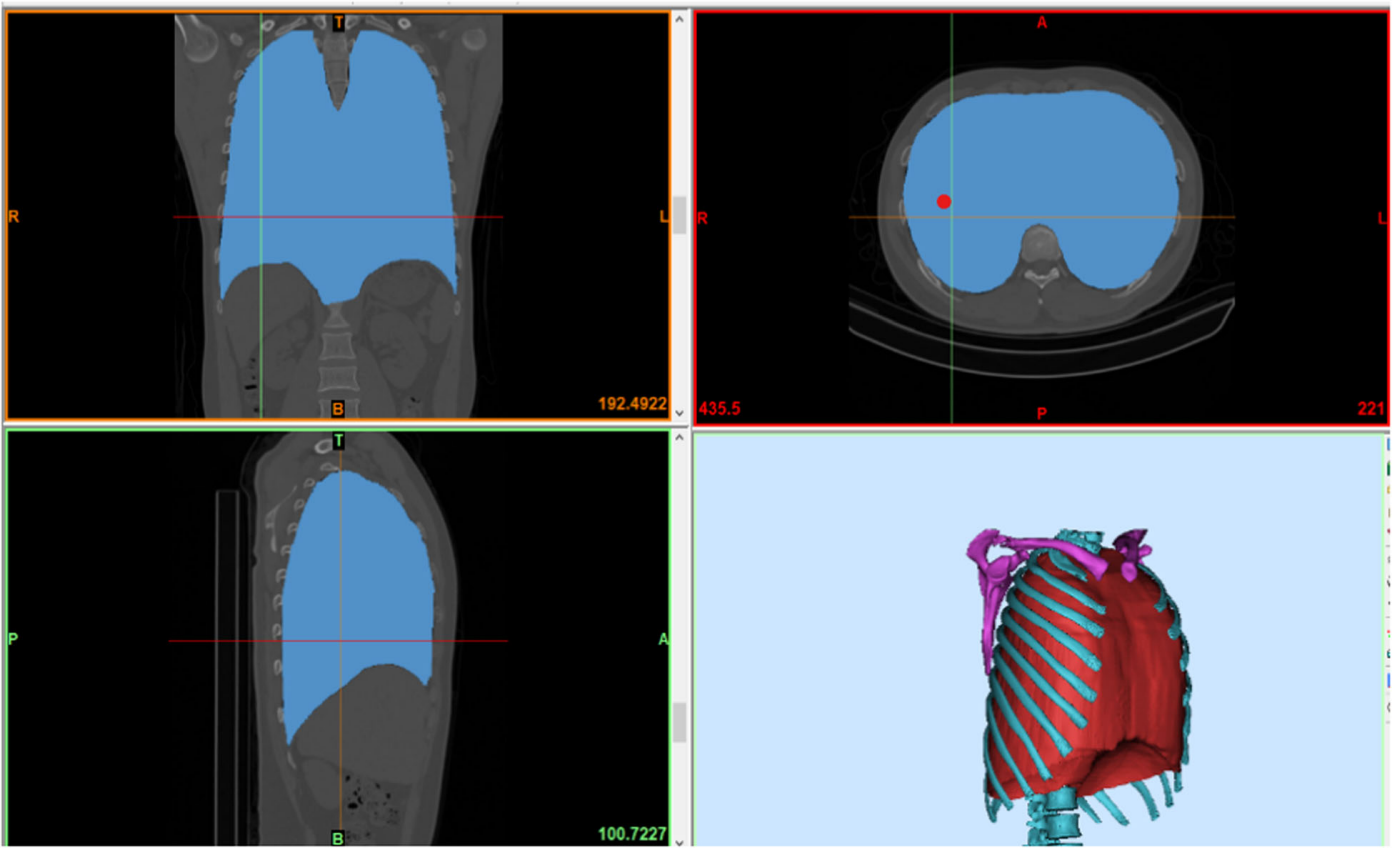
This normal thoracic model was reconstructed based on the chest CT data of a 27-year old man by using Mimics software
